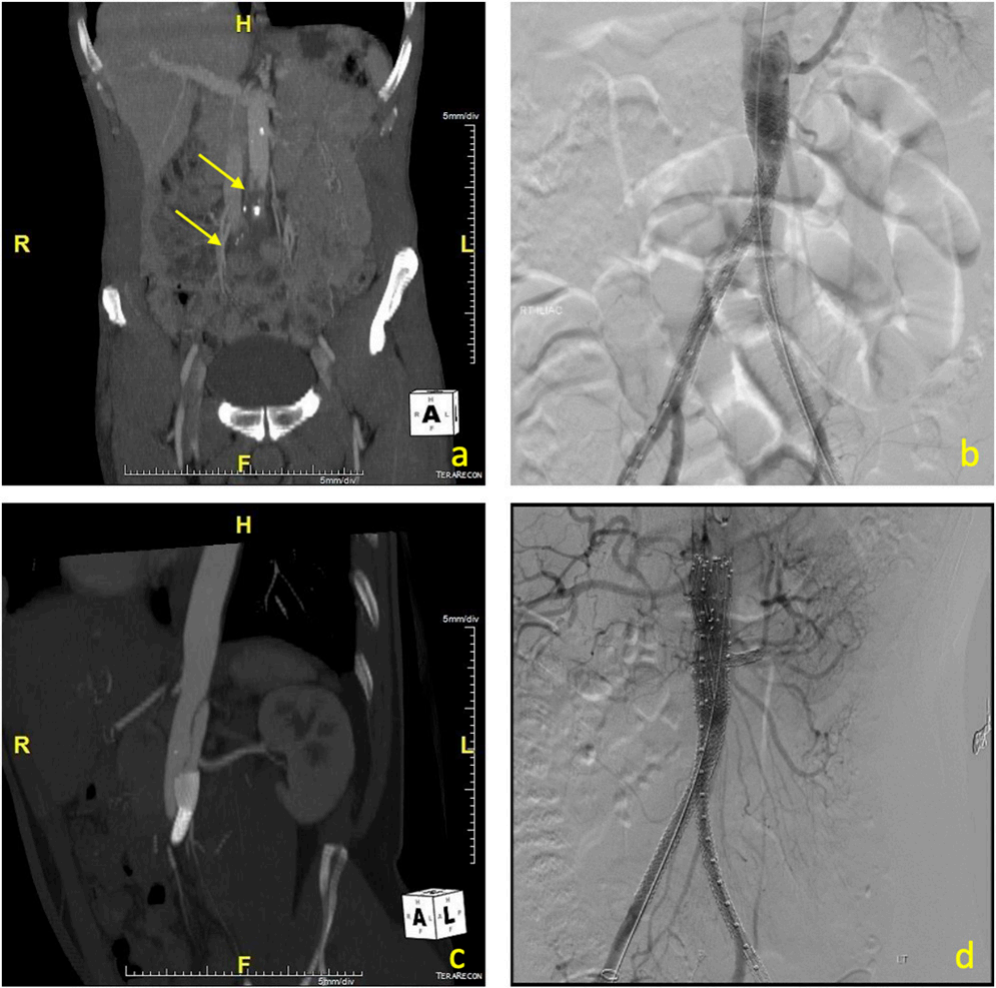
(a) CT angiogram of the abdomen showing occlusion of the aortic bifurcation (yellow arrows), (b) aortoiliac reconstruction with the CERAB procedure, note of quite low origin of left renal artery, (c) follow-up CT angiogram, showing proximal flow limiting dissection of the aorta above the CERAB procedure, (d) proximal extension with custom-made fenestrated cuff with one fenestration for the left renal artery and one scallop for the right renal artery (combined CERAB and fenestrated graft).
